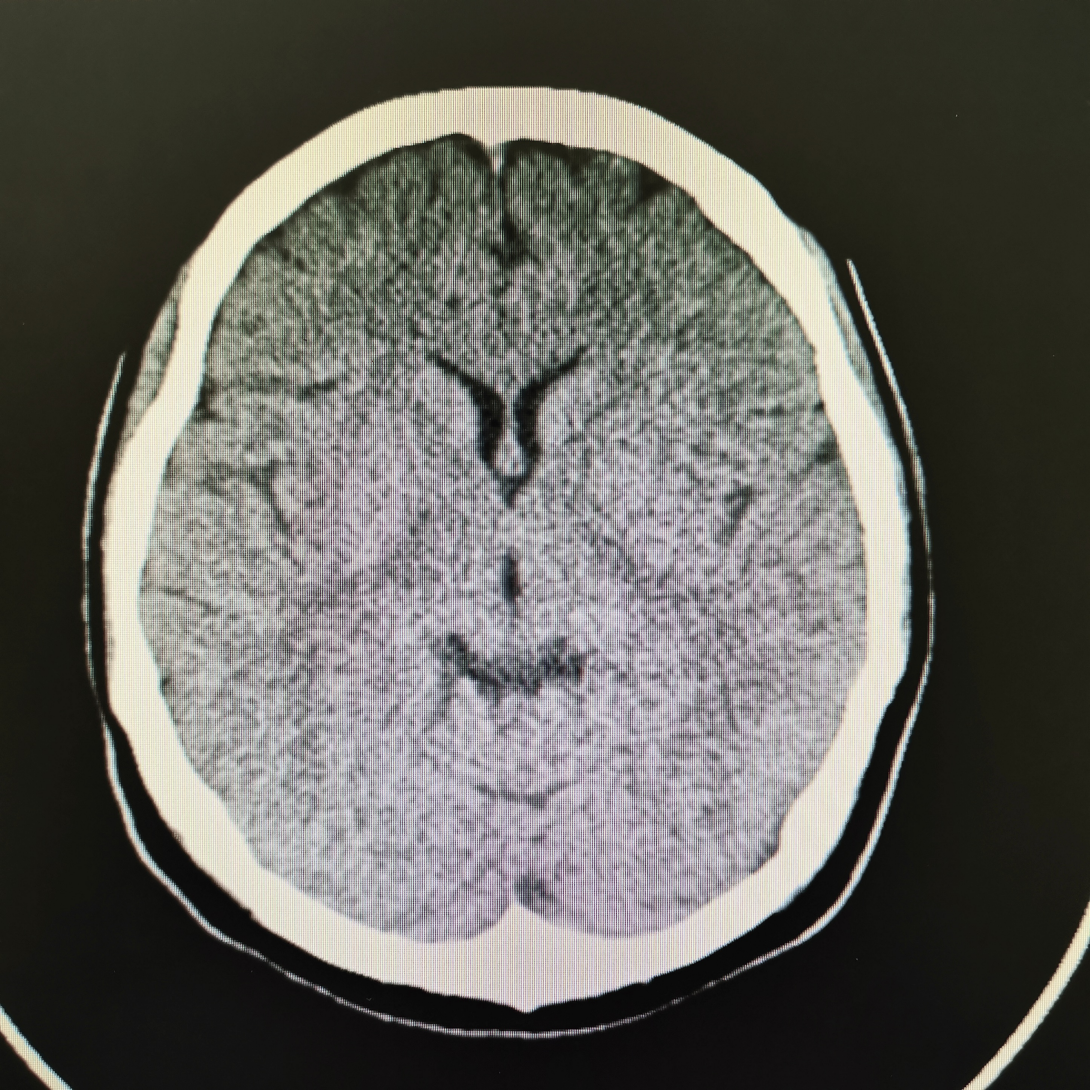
CT brain (axial view) shows no significant abnormalities
